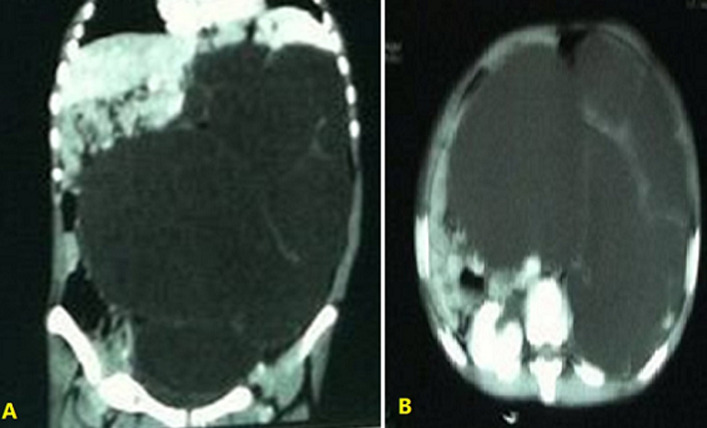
abdominal computed tomography (CT) scan relived giant hydronephrosis of the left kidney: A) coronal views; B) axial views
